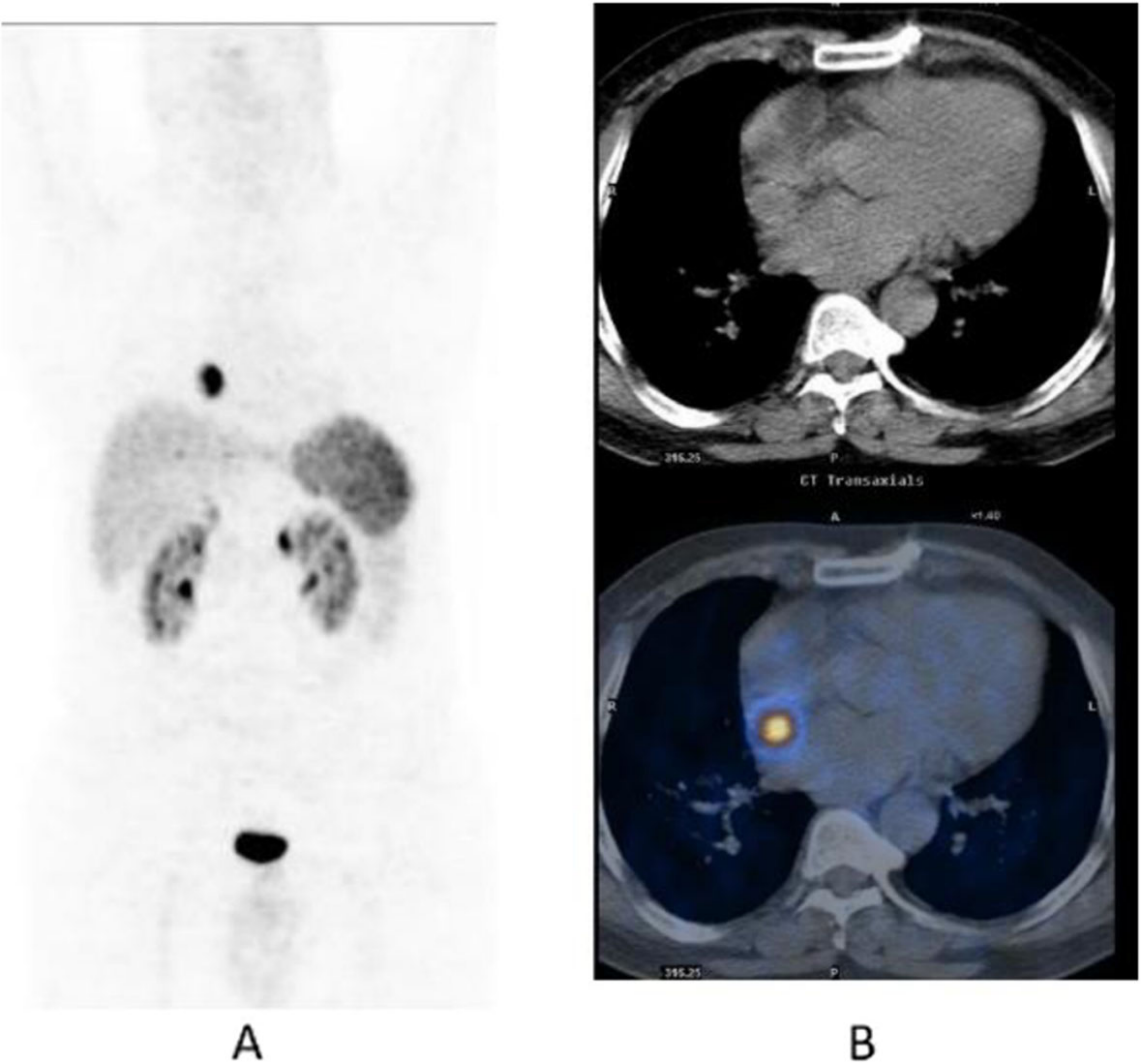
^68^Ga-DOTA peptide, suspected recurrence of paraganglioma. *Clinical history*: 39 y.o. man with a previous history of paragangliomas. During follow-up CT suspected a relapse in the thorax. *PET/CT findings*: intense focal uptake in a para-caval round shaped lesion (a MIP, b CT and fused PET/CT) consistent with a paraganglioma
